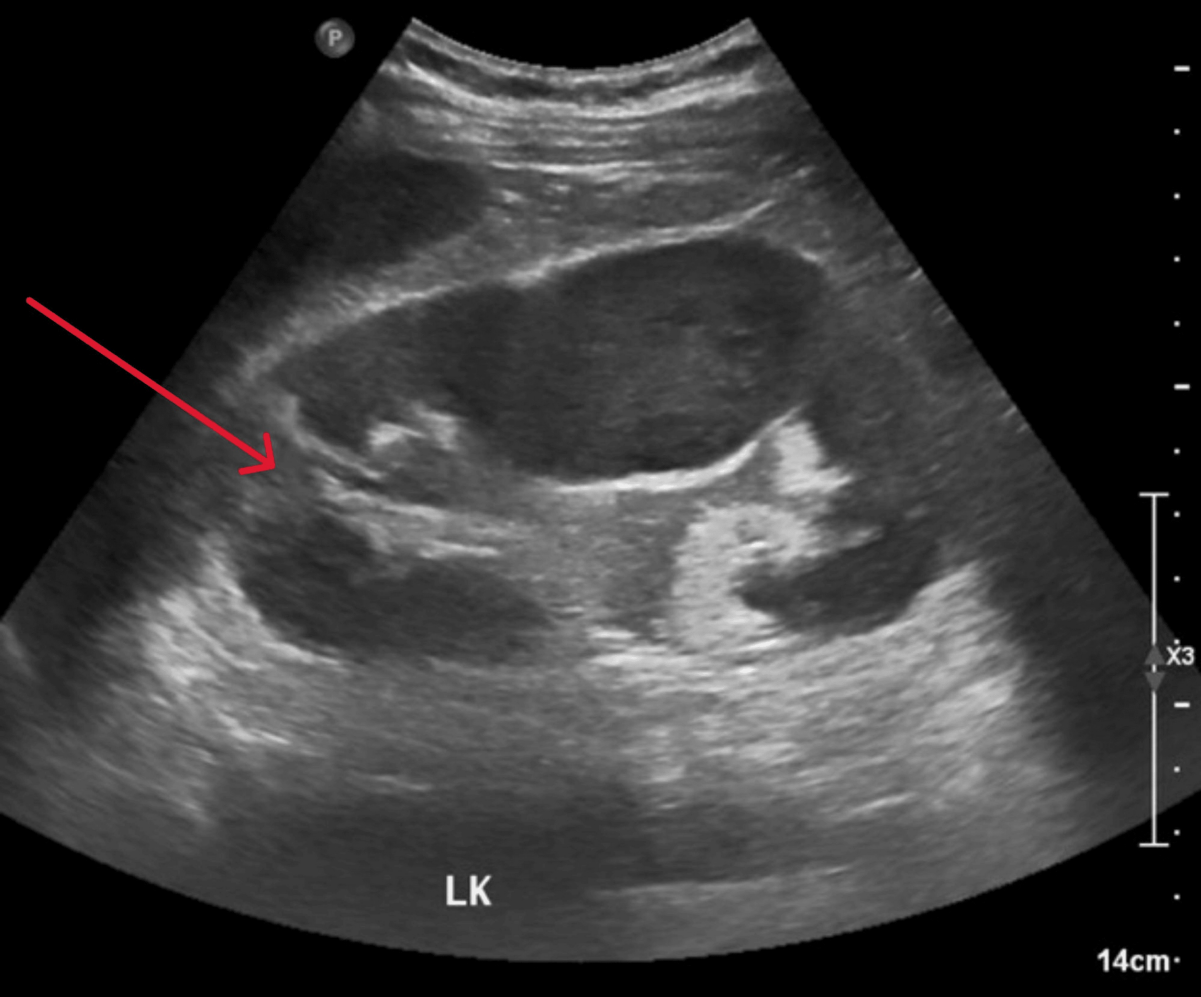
Kidney ultrasound revealed mild renal pelvic dilatation with internal echogenic debris (red arrow)
